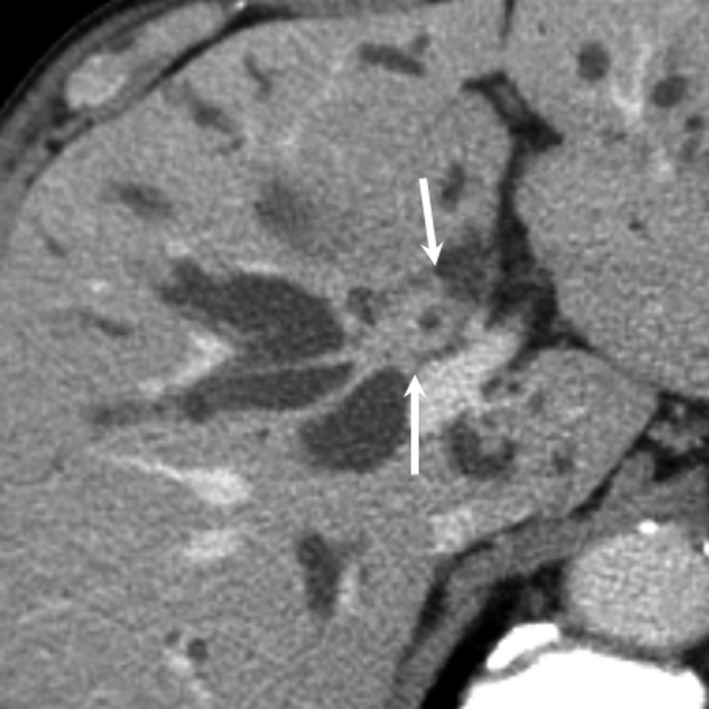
CT image of IgG4‐SC. Contrast‐enhanced CT (delayed image) demonstrates concentric wall thickening of the hilar bile duct (arrows)
